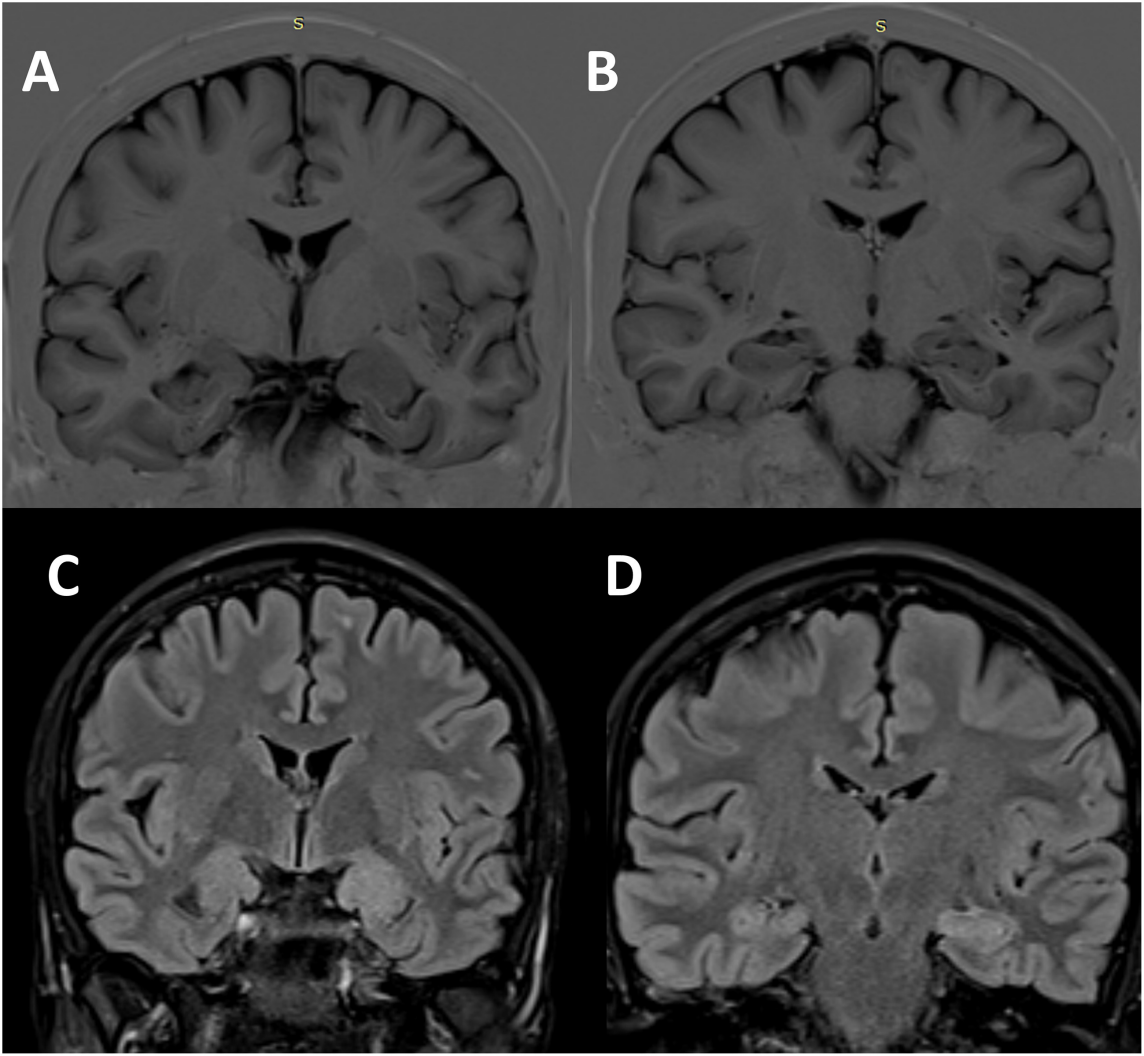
Structural brain imaging in Case 2. Interictal cerebral MRI scan—coronal sections—showing the enlargement of the left amygdala (A) and hippocampus (B) on T1-inversion recovery pulse sequence, as well as FLAIR hyperintensities in the same regions [(C) amygdala; (D) hippocampus].
